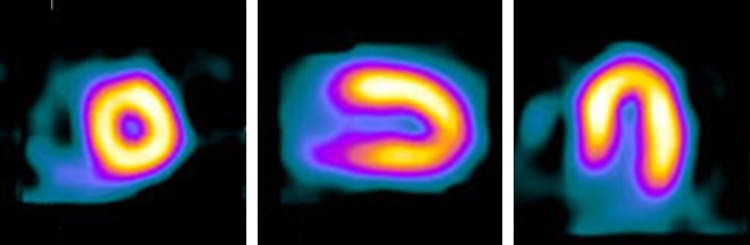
Normal single photon emission computed tomography examination: Left ventricle seen in short axis, vertical long axis, and horizontal long axis. Adapted from Hasegawa *et al.*^142^
